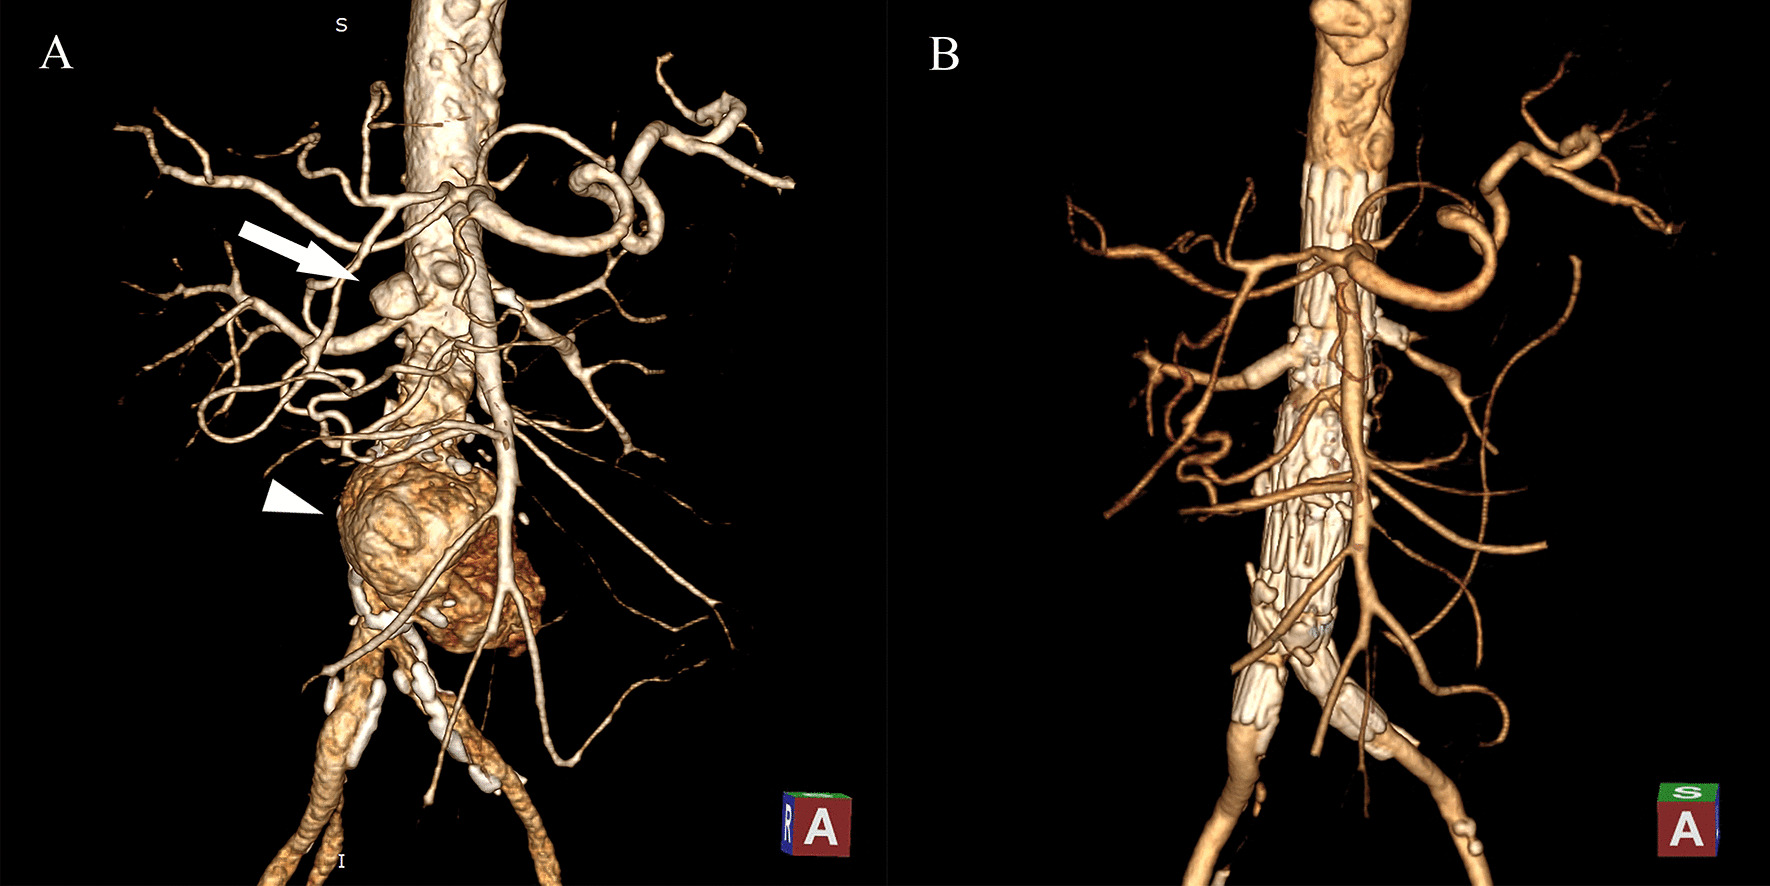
CT scans of the first patient. A Preoperative CT showed the renal aneurysm (white arrow) and the aortic aneurysm (white arrowhead). B 6-month follow-up CT scans
